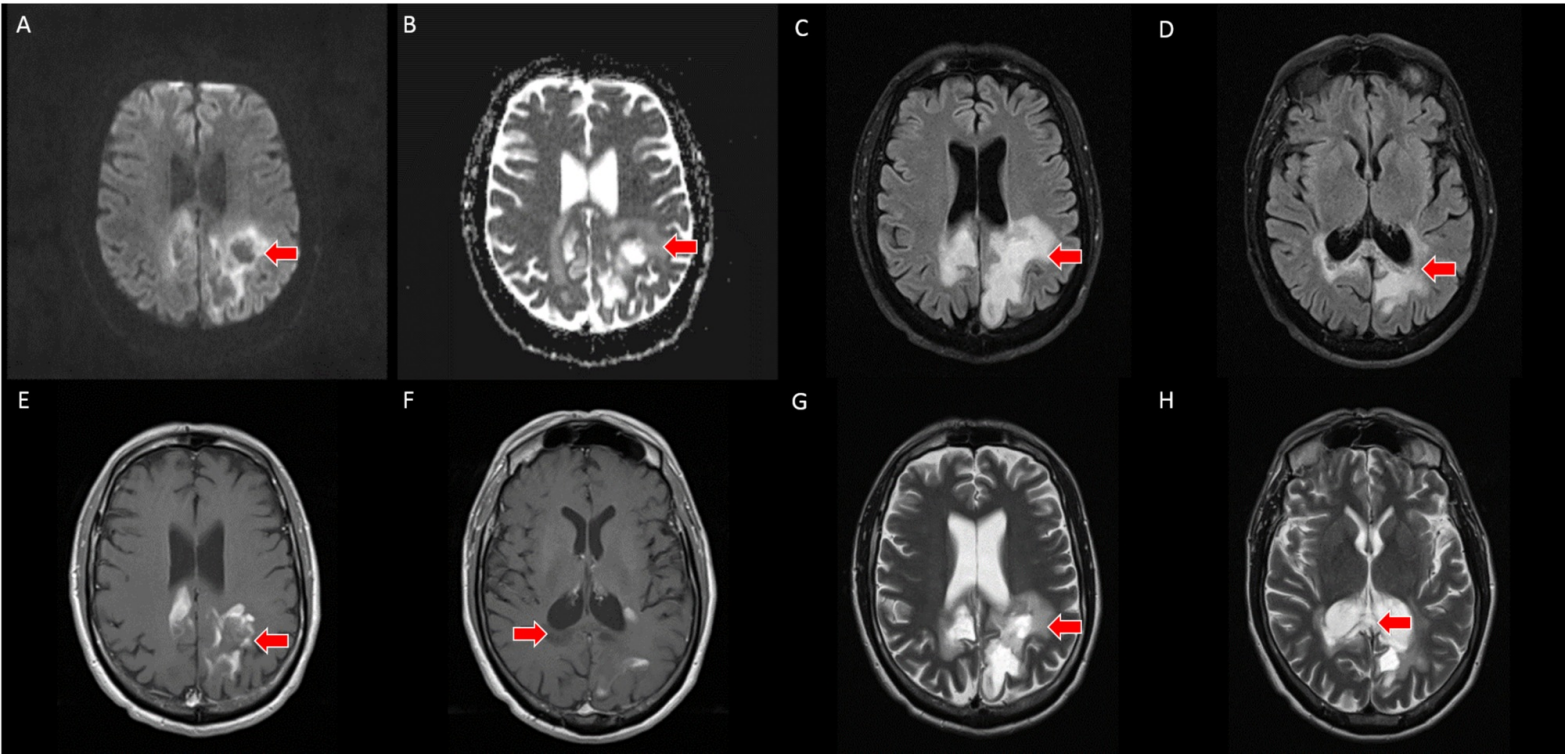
A, B - axial slices from three-month follow up brain MRI with provided DWI/ADC sequences (top left) demonstrate T2 shine through without restricted water diffusion (red arrows) in the region of previously seen restricted water diffusion. C, D - axial slices from three-month follow up brain MRI with FLAIR sequences demonstrate necrosis and cystic change within the corpus callosum and subcortical white matter of the bilateral parietal lobes (red arrows). E, F - axial slices from three-month follow up T1-weighted post-contrast brain MRI demonstrate resolution of the homogeneous enhancement with an incomplete, peripheral enhancement pattern. G, H - axial slices from three-month follow up brain MRI with T2-weighting demonstrate necrosis and cystic change within the corpus callosum and subcortical white matter of the bilateral parietal lobes (red arrows) MRI: magnetic resonance imaging, DWI: diffusion-weighted imaging, ADC: apparent diffusion coefficient, FLAIR: fluid-attenuated inversion recovery.
